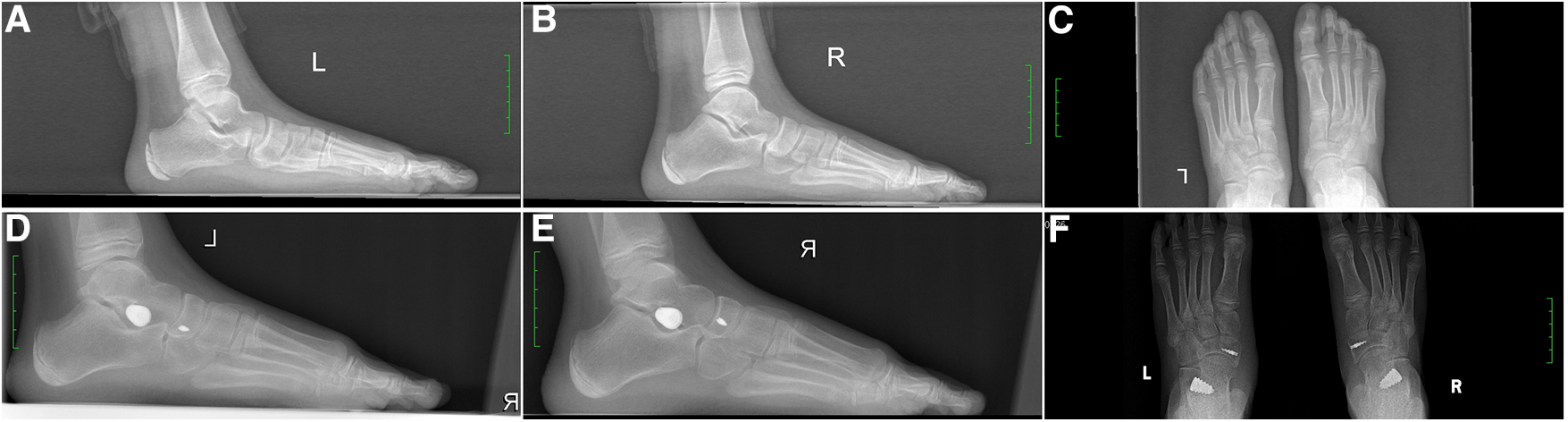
An 11-year-old male patient with bilateral type II painful accessory navicular (AN) combined with flexible flatfoot underwent a modified Kidner surgery combined with subtalar arthroereisis tarsal screw implantation. (A) Lateral x-ray images of left feet before surgery: Meary's angle: 16, APTMT: 14, Talar-second metatarsal angle: 19, Pitch angle: 15, TCA: 52, Calcaneal angle: 51, Talar tilt angle: 35, LTCA: 16. (B) Lateral x-ray images of right feet before surgery: Meary's angle: 16, APTMT: 11, Talar-second metatarsal angle: 13, Pitch angle: 17, TCA: 51, Calcaneal angle: 51, Talar tilt angle: 33, LTCA: 18. (C) AP x-ray images of both feet before surgery, (D) Lateral x-ray images of left feet 1-year postoperative follow-up: Meary's angle: 4, APTMT: 3, Talar-second metatarsal angle: 3, Pitch angle: 17, TCA: 36, Calcaneal angle: 49, Talar tilt angle: 24, LTCA: 9. Parameters shows good positioning of the anchor screw and tarsal screw and good alignment of foot arch. (E) Lateral x-ray images of right feet 1-year postoperative follow-up: Meary's angle: 4, APTMT: 1, Talar-second metatarsal angle: 3, Pitch angle: 18, TCA: 42, Calcaneal angle: 46, Talar tilt angle: 23, LTCA: 8. Parameters shows good positioning of the anchor screw and tarsal screw and good alignment of foot arch. (F) AP x-ray images of both feet 1-year postoperative follow-up, showing correction of AN deformity in both feet.
